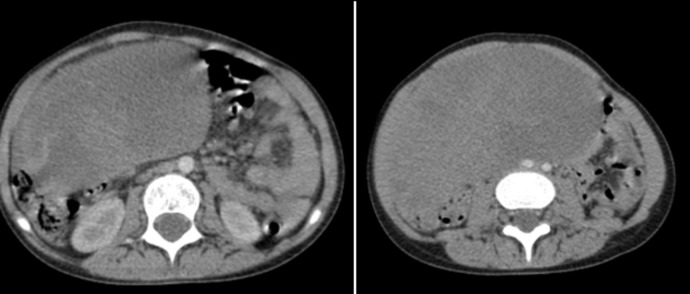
the axial section of a pelvic computed tomography scan showing a right ovarian mass
